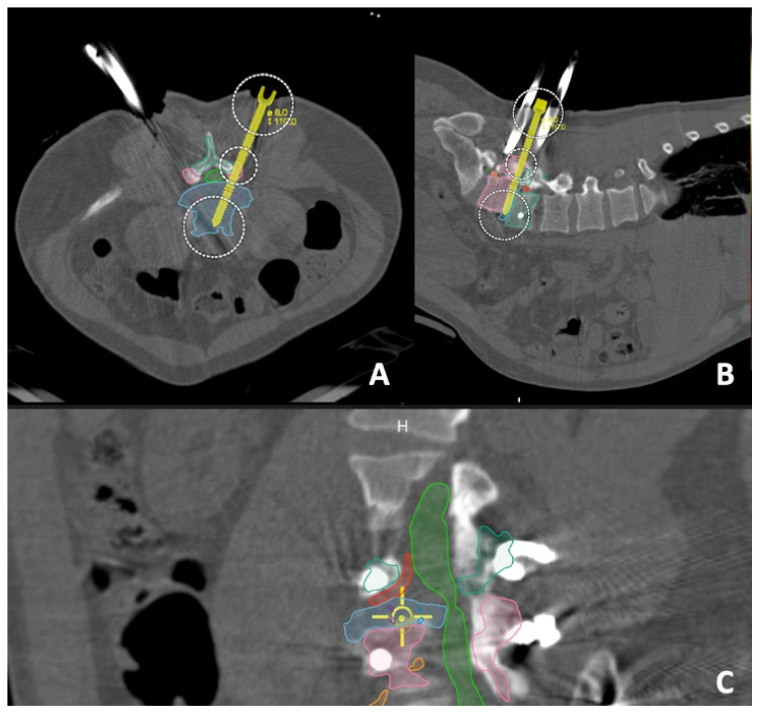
(A) Axial, (B) sagittal and (C) bird’s eye views of the merging of the preoperative planning with the intraoperative CT scan assuring the accuracy and confirming the safety of our corridor, modelled by an elongated screw, before advancing with the steps of interbody fusion.
